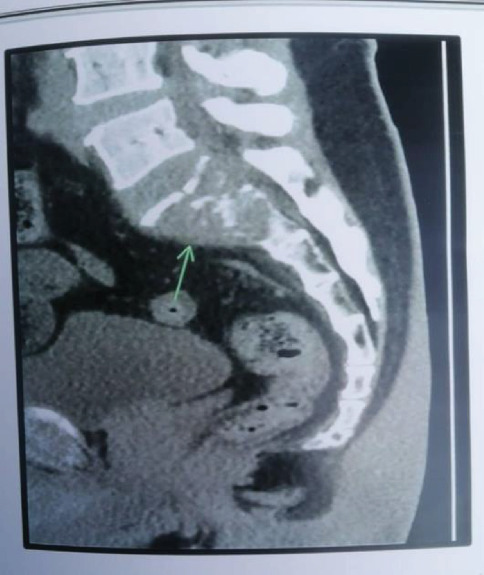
CT spine. S1–S2 destructive lesion.
